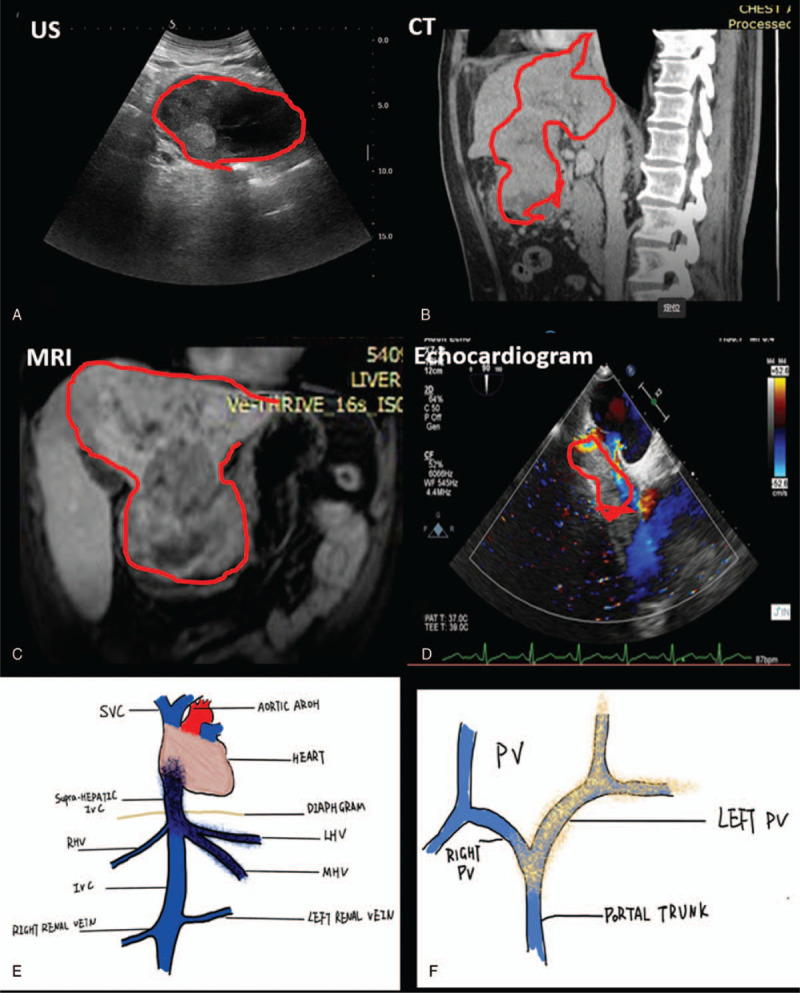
Preoperative assessment of the HCC lesion. A, ultrasonography imaging; B, CT scan showed a giant HCC lesion with tumor thrombi extending into right atrium; C, MRI scan showed huge mass in left lobe; D, echocardiogram showed a 5.0 cm × 2.8 cm mobile mass in supra-hepatic IVC and right atrium; E, the extension of tumor thrombi in IVC, hepatic veins and right atrium; F, the extension of tumor thrombi into portal vein.
